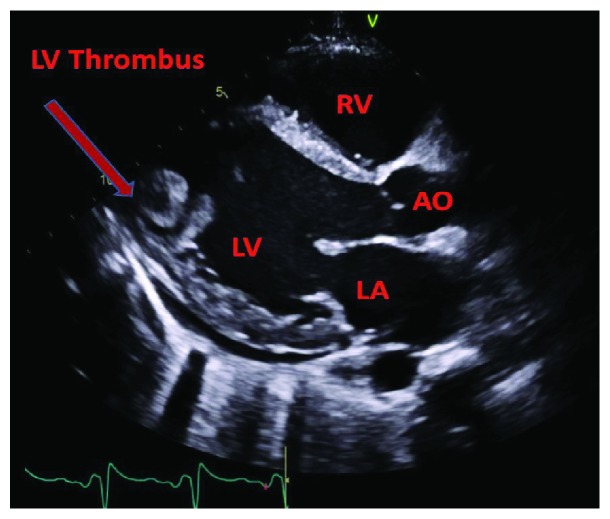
Parasternal long axis view showing LV thrombus. LA: left atrium; AO: aorta; RV: right ventricle; LV: left ventricle.
